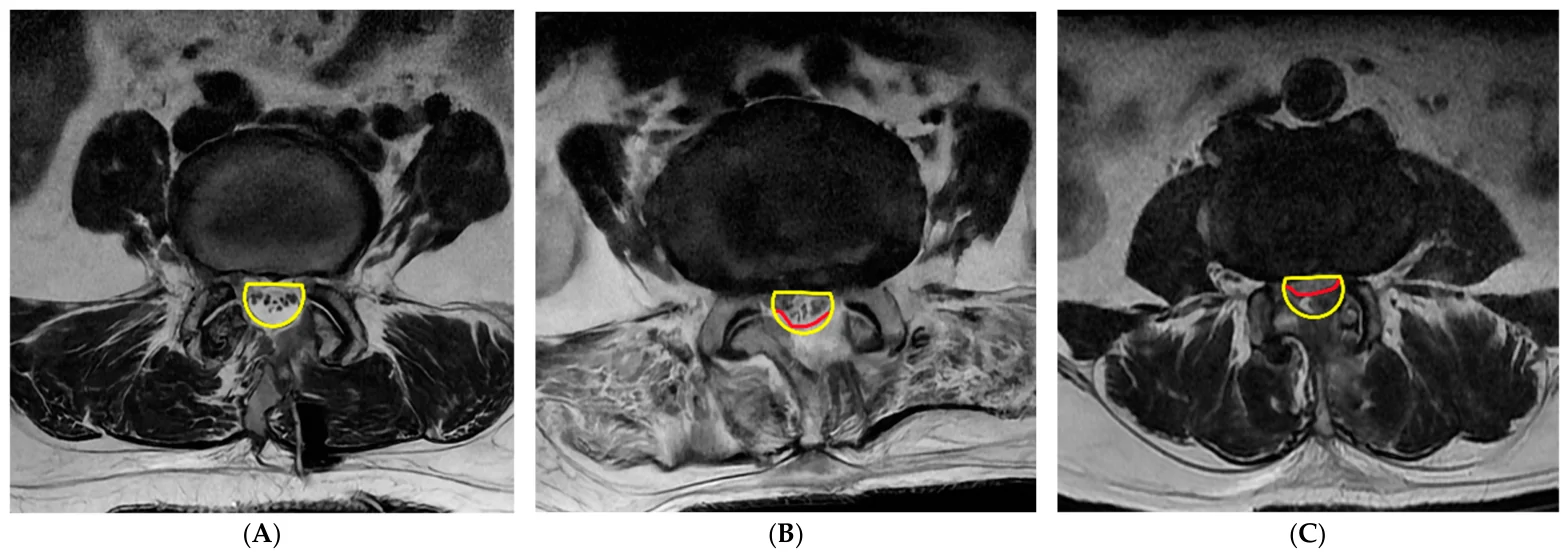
Postoperative spinal epidural hematoma grading system on axial T2-weighted MRI. (A) Grade 0, no hematoma. (B) Grade I, hematoma causing <25% compression of the spinal canal. (C) Grade III, hematoma causing 50–75% compression of the spinal canal. The yellow outlines indicate the margins of the thecal sac, and the red lines delineate the boundary between the hematoma and the thecal sac.
